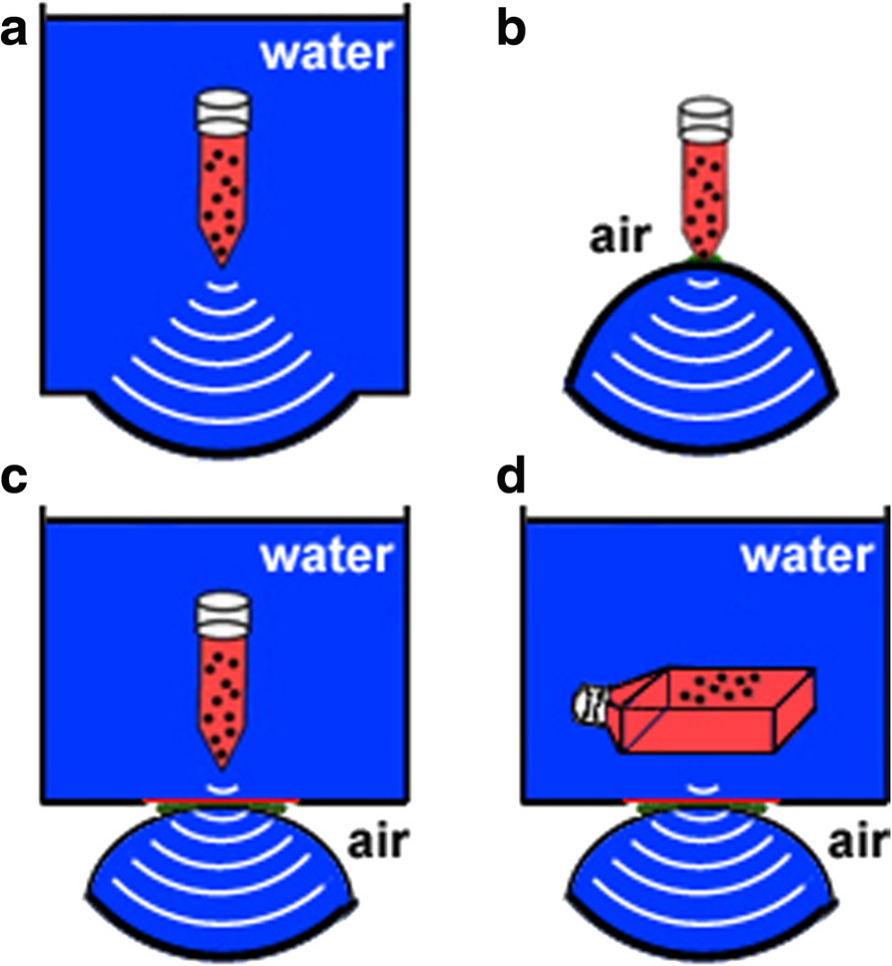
The mainly used setups for in vitro shockwave experiments: tube with single cell suspension in a water bath coupled to a shockwave transducer by water (a), tube with single cell suspension coupled to a shockwave transducer with coupling gel and surrounded by air (b), ultrasound gel coupling of the transducer to a water basin with a membrane, and either a tube with cell suspension (c) or a cell culture flask with adherent cells (d)
